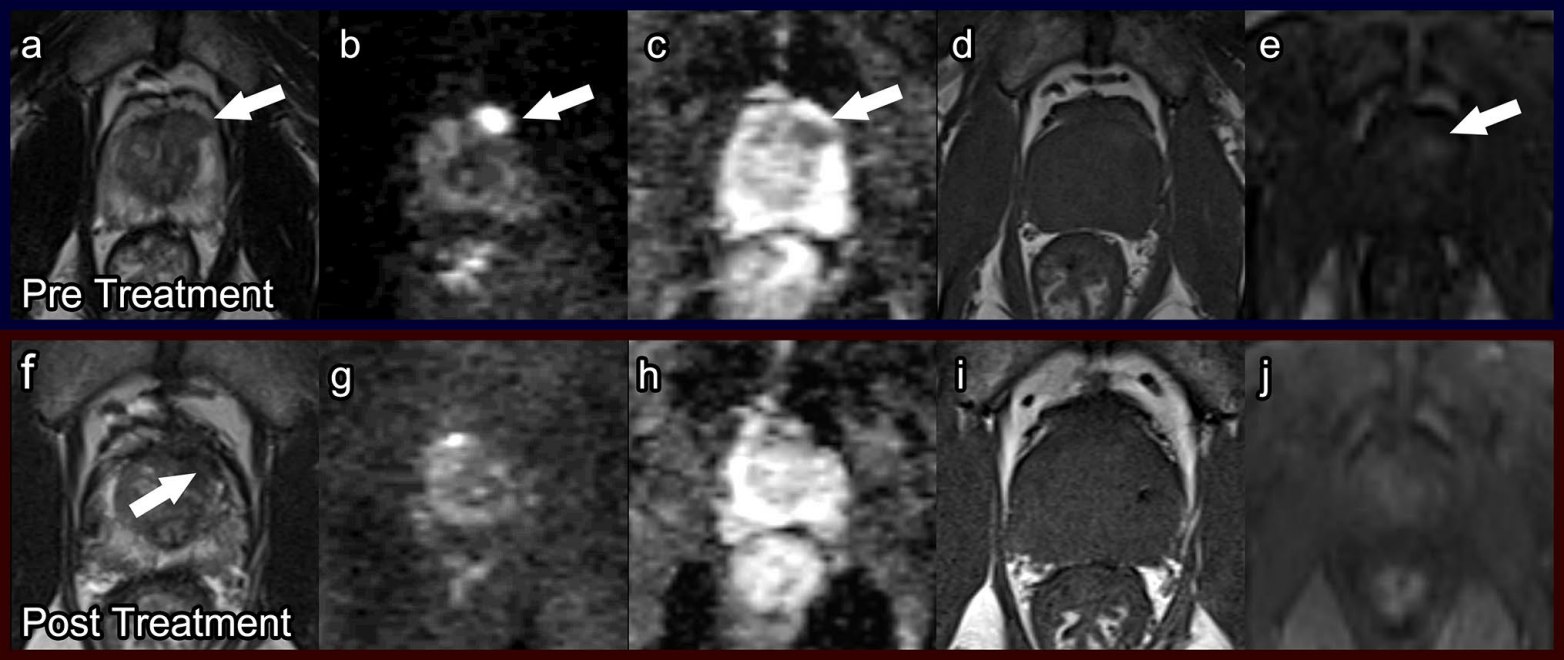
Cryotherapy. Pre-treatment prostate MRI: Axial T2 (a), DWI (b), ADC (c), pre-contrast T1 (d), and post-contrast T1 (e) demonstrate a PI-RADS 5 lesion (arrows) in the left mid-anterolateral peripheral zone. Biopsied as Gleason score 3 + 4 prostate cancer. Post-cryoablation prostate MRI at 1 year: Axial T2 (f), DWI (g), ADC (h), pre-contrast T1 (i), and post-contrast T1 (j) demonstrate ovoid T2 dark scarring (arrow), mild prostatic atrophy without restricted diffusion or hyperenhancement
